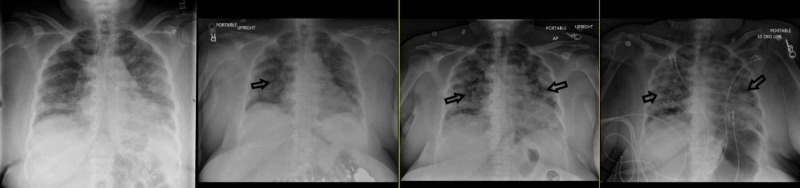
Chest x-ray progression, left to right, showing worsening diffuse bilateral patchy opacities (black arrows) with progressive obscuration of the cardiac silhouette.
